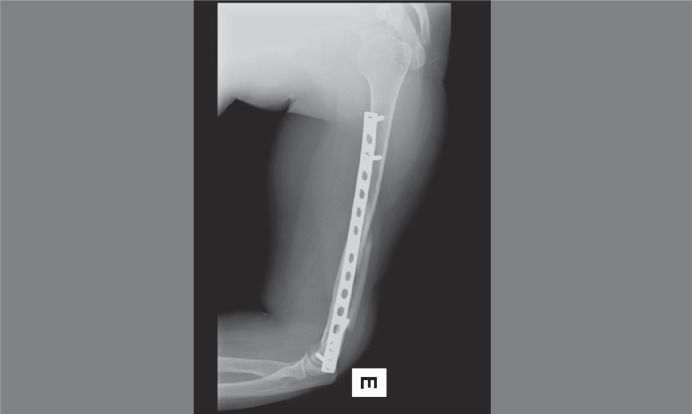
Postoperative X-Ray (Profile) of patient treated with MIPO technique and direct visualization of the radial nerve.
